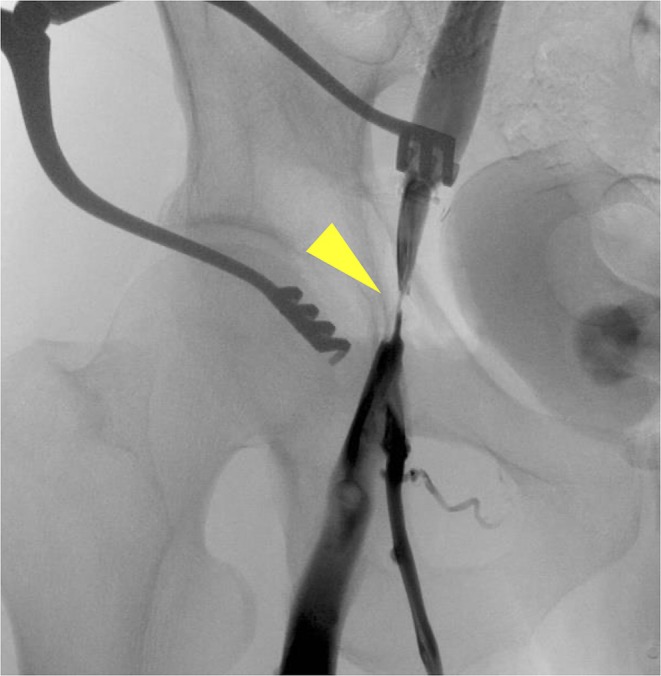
The stenosis of the right femoral vein due to Perclose. One week after surgery, the patient developed right lower leg edema. Contrast‐enhanced computed tomography revealed 99% stenosis of the femoral vein. Surgical exploration via femoral incision confirmed venous ligation caused by the Perclose device, which was subsequently released.
